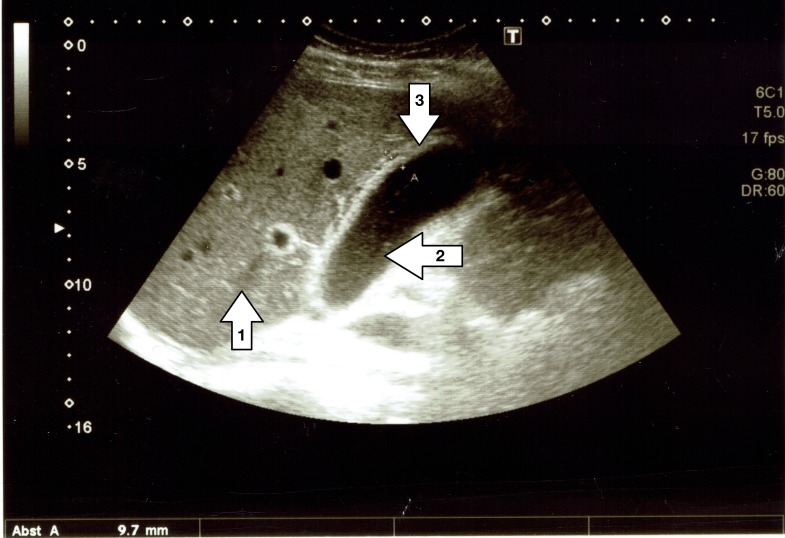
Ultrasound image of patient's gallbladder The figure shows the patient's liver (arrow 1) and gallbladder (arrow 2) on admission day. While no stones can be found inside, the gallbladder wall (arrow 3) presents as multilayered and thickened (9.7 mm).
